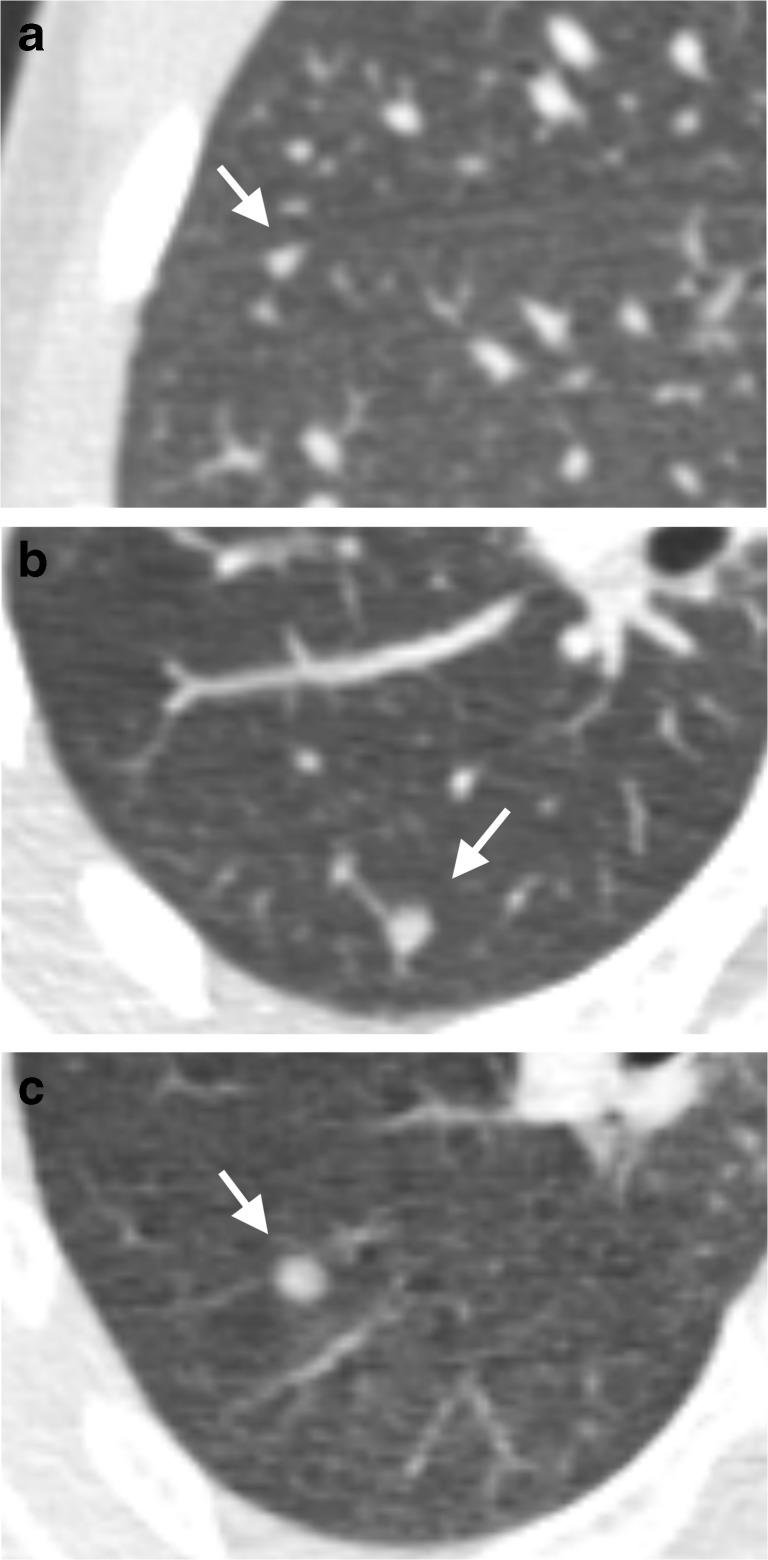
Axial 0.9-mm-thick CT images show examples from the perifissural nodule classification [14]. a A 3-mm typical perifissural nodule (*arrow;* fissure attached, homogenous, smooth margins and triangular shape) in an 8-year-old boy. b A 5-mm atypical perifissural nodule (*arrow;* fissure attached, homogenous, smooth margins and convex on one side, rounded on the other) in an 11-year-old boy. c A 5-mm non–perifissural nodule (*arrow;* fissure attached, inhomogenous, blurred margins and spherical shape) in a 12-year-old boy
